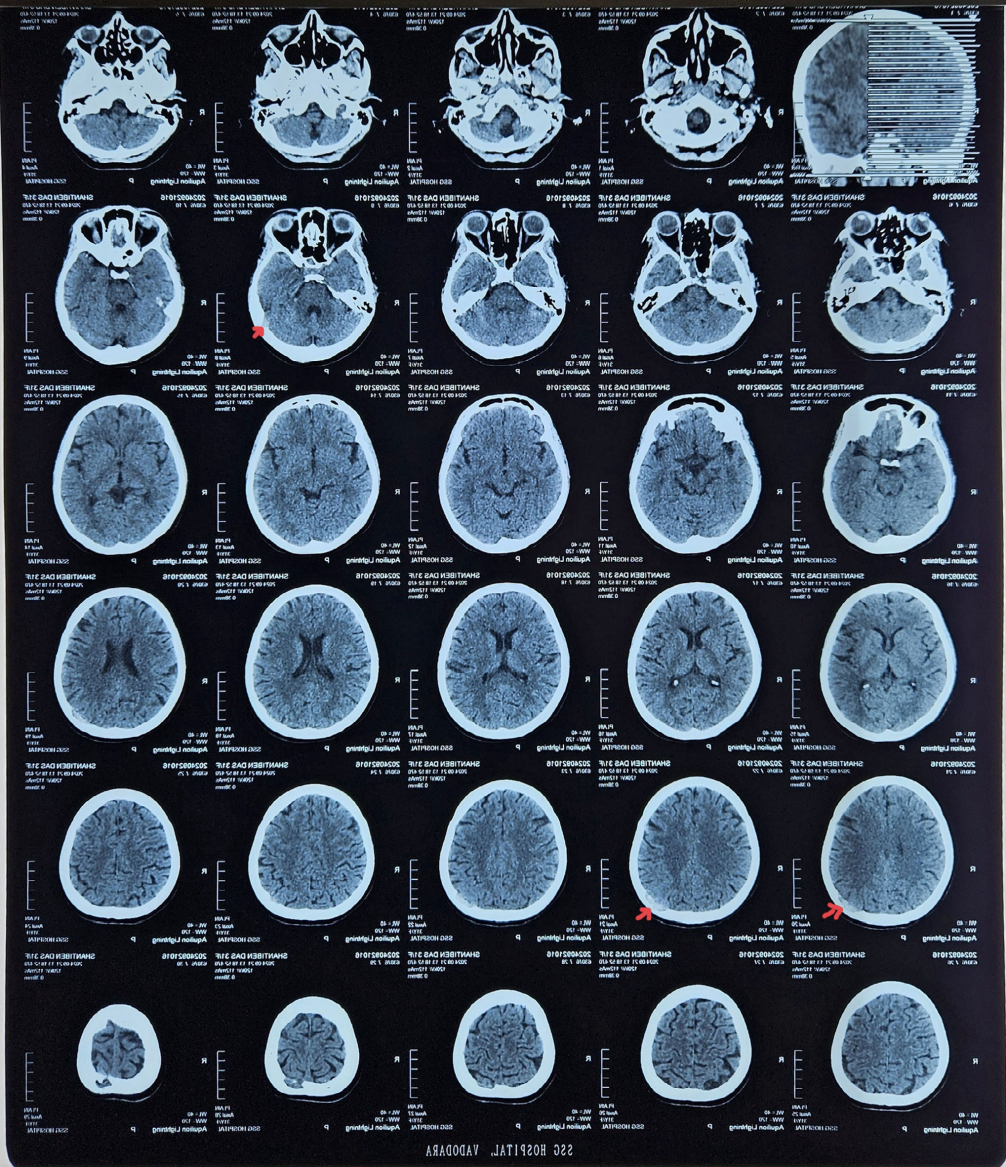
CT scan of head showing lytic lesion as indicated by the red arrow.
